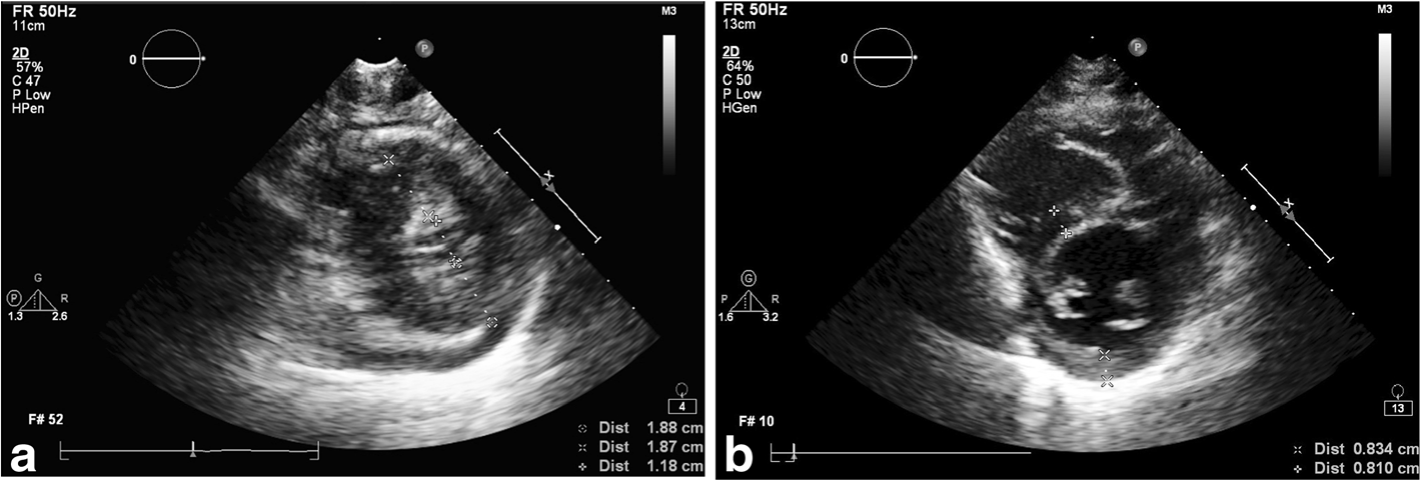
a. Echocardiogram after cardiac arrest during the second presentation. Left ventricular wall thickening with underfilled left ventricle. b. Echocardiogram 7 days after the second presentation. Left ventricular wall thickening normalised
